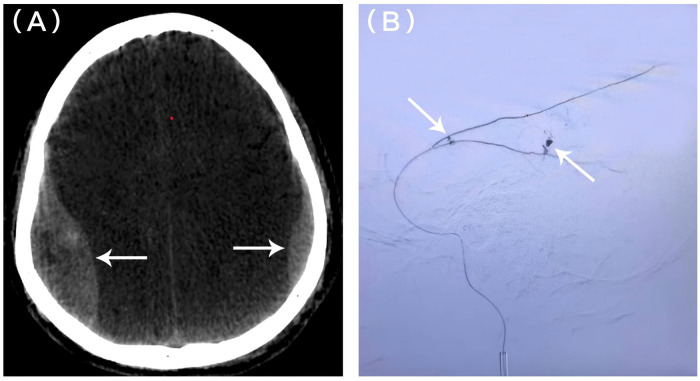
Interval development of contralateral EDH detected after the initial embolization. (A) Axial noncontrast CT image obtained after the initial embolization showing a newly developed epidural hematoma in the right parietal region (arrow). (B) Digital subtraction angiographic image of the right middle meningeal artery (MMA) demonstrating dural arterial supply to the contralateral (right) epidural hematoma (arrow).
